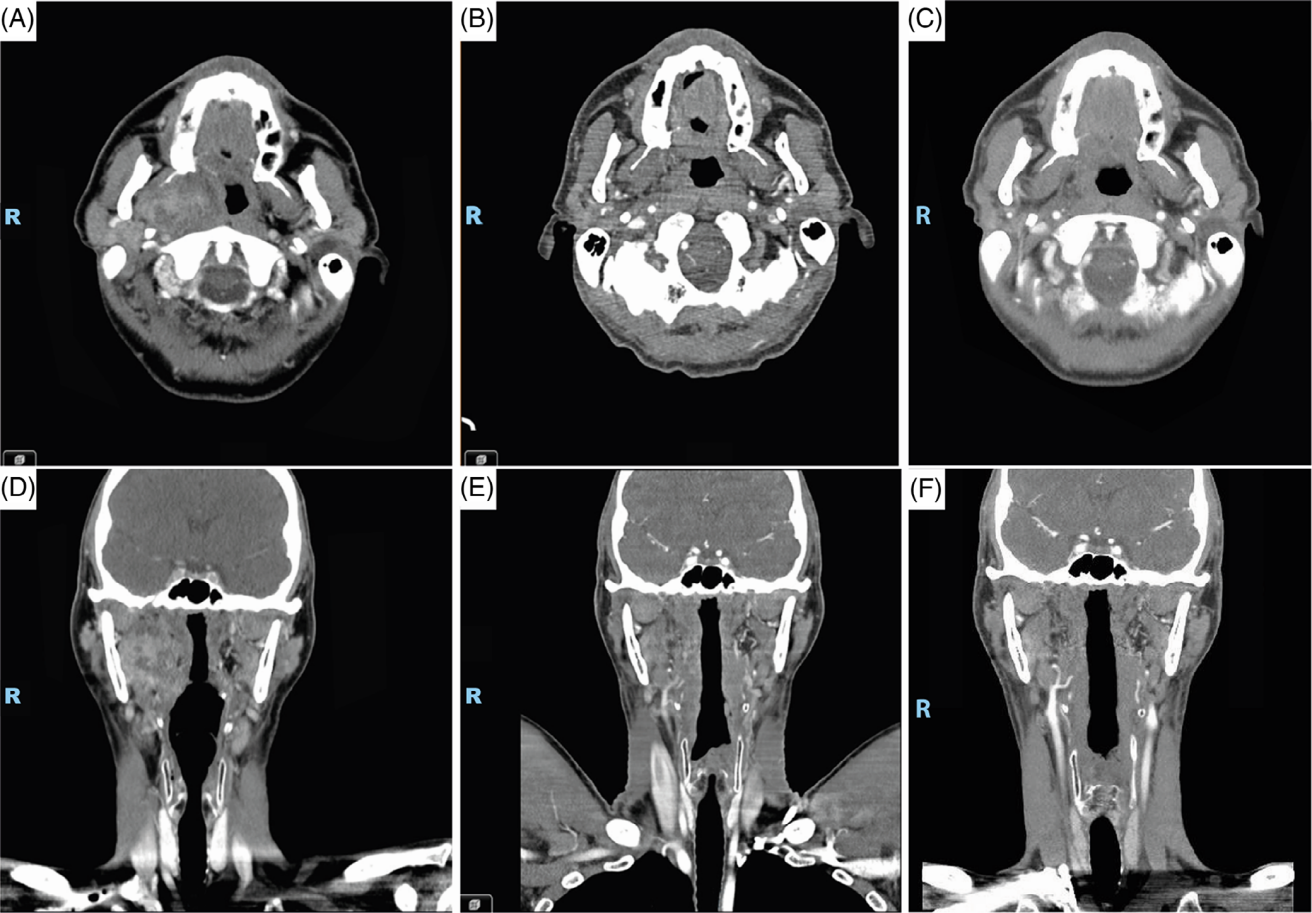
Pre‐surgical CT scan. 40 × 29 mm lesion in the right parapharyngeal space: (A) axial and (D) coronal images. Post‐surgical CT scan, follow‐up after 8 weeks of treatment: (B) axial and (E) coronal images. Follow‐up after 7 months of treatment: (C) axial and (F) coronal images
